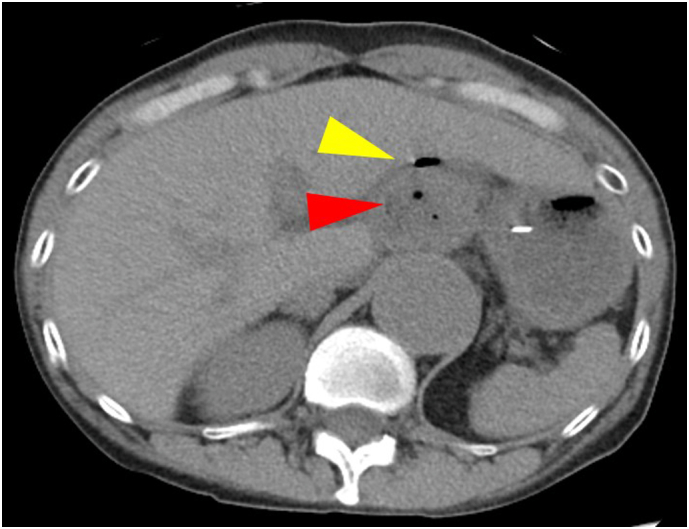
Computed tomography findings on the third day after induction of chemotherapy. Free air in the abdominal cavity (yellow arrowhead) and necrosis in lymph node metastasis in the lesser curvature side of the stomach (red arrowhead) were observed.
